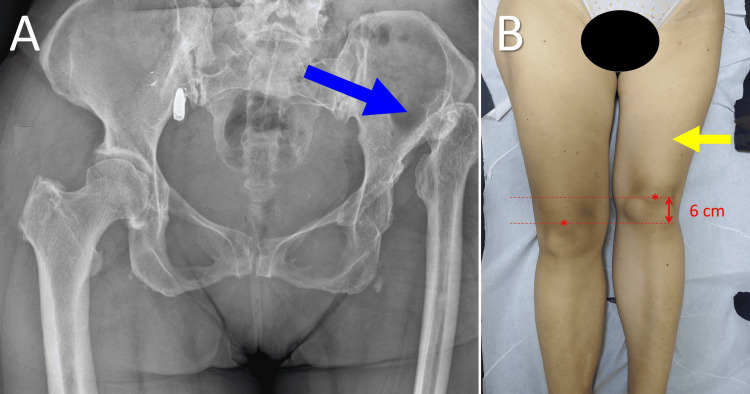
Neglected high dislocation of the left hip in a 52-year-old woman A. An anteroposterior X-ray of the pelvis indicating high dislocation of the left hip with a false acetabulum (blue arrow); B. No asymmetric skin fold is evident on the 6 cm shorter left thigh (yellow arrow; asterisks indicate the center of the patellae). Image credits: P. Samelis
